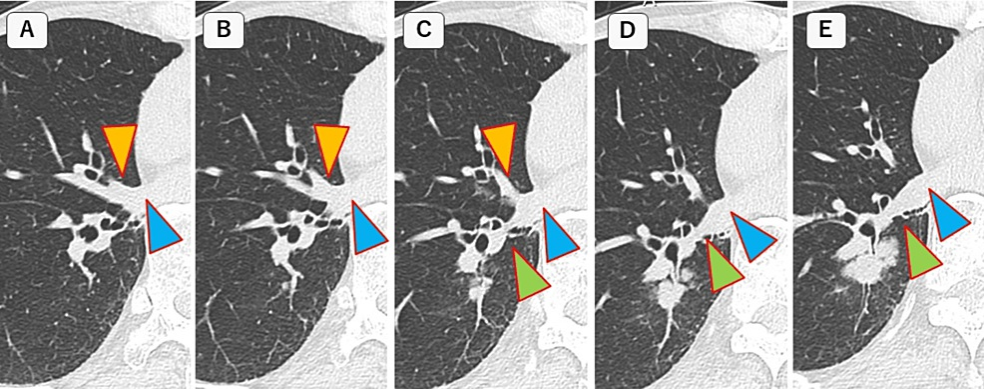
CT findings before surgery (A-E) Serial CT image with a 1 mm thick slap from cephalad to caudal. The yellow arrow indicates the middle lobe pulmonary vein (PV), the green arrow shows the lower lobe PV, and the blue arrow shows the inferior PV. The middle lobe PV joins the inferior PV. However, these images did not show the posterior segmental vein of the upper lobe (V^2^) merging into the inferior PV CT: computed tomography
